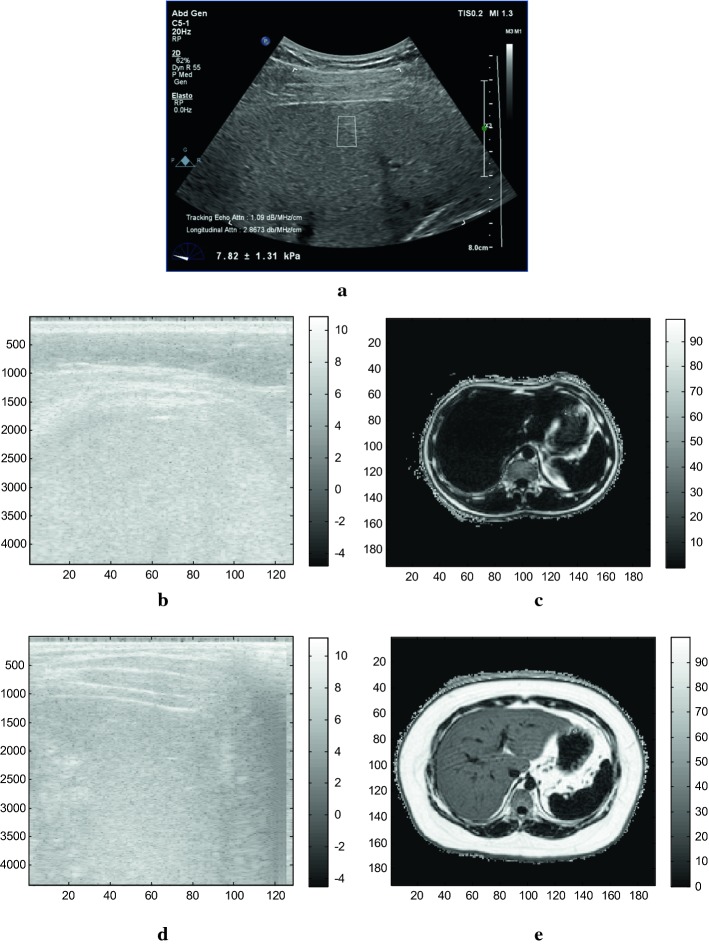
Demonstration of the acquired data in the study. a denotes the interface of the ultrasound data acquisition based on the designed ultrasound sequence. b, d The direct logarithmic results of the envelope of the corresponding raw radiofrequency ultrasound data. c, e The MRI fat quantification results. b, c are from the patient with liver fat fraction as 2.56%. d, e are from the patient with liver fat fraction as 29.45%. The scale bar in ultrasound images denotes the intensity and the one in MRI images denotes the percentage of fat fraction
